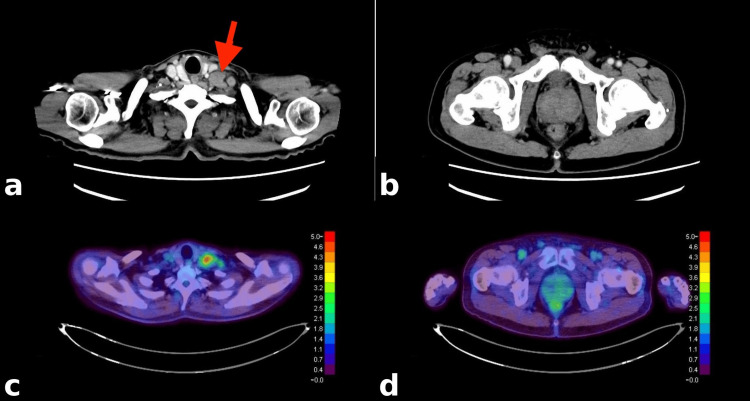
Pretreatment CT and FDG-PET images. (a and c) Contrast-enhanced CT and FDG-PET images of a metastatic cervical lymph node (red arrow), demonstrating increased FDG uptake (SUVmax: 5.3). (b and d) Contrast-enhanced CT and FDG-PET images of the prostate gland, showing no abnormal FDG uptake. CT, computed tomography; FDG-PET, 18F-fluorodeoxyglucose positron emission tomography; SUVmax, maximum standardized uptake value
